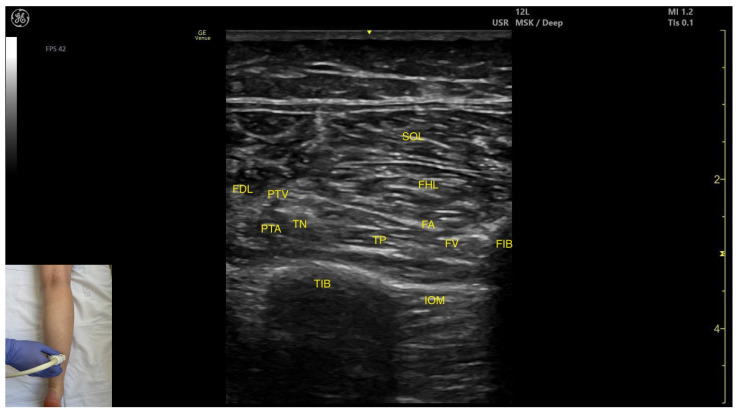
Ultrasound anatomy of the tibialis posterior—posterior window: FDL—flexor digitorum longus; PTA—posterior tibial artery; PTV—posterior tibial vein; TN—tibial nerve; TIB—tibia; TP—tibialis posterior; SOL—soleus; FHL—flexor hallucis longus; FA—fibular artery; FV—fibular vein; IOM—interosseous membrane; FIB—fibula.
